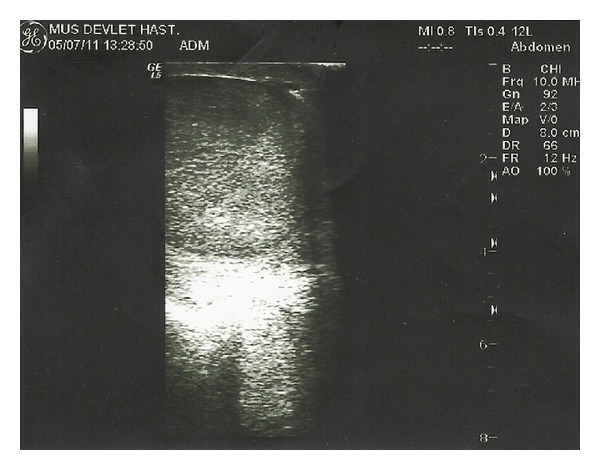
The scrotal color Doppler US image of a patient with BEO.
